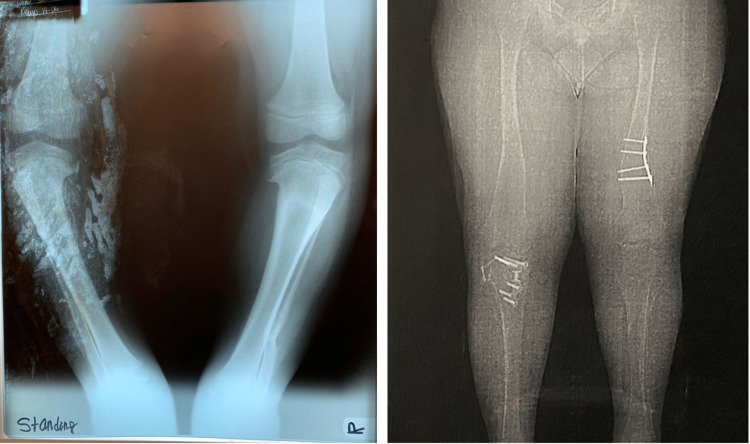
X-ray of case 1 before surgical intervention (left) versus CT scanogram after surgical intervention (right).
